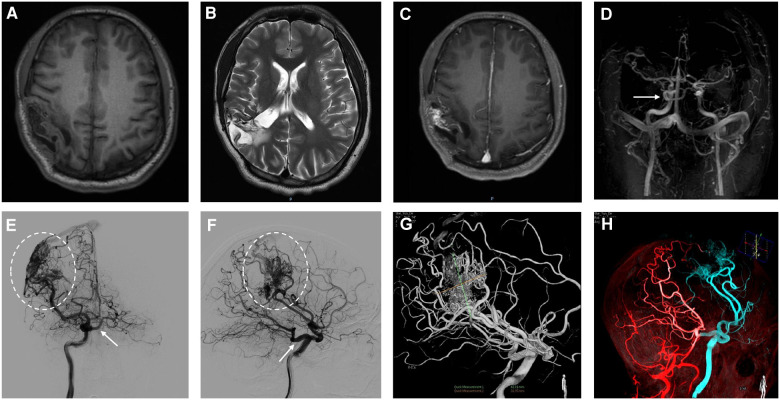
In the right parietotemporal lobe, an irregularly circular space-occupying lesion was found with slightly long T1 shadows (A). T2-weighted image shows a parietotemporal lesion with mixed signal characteristics (B). Enhanced MRI shows heterogeneous enhancement in the right parietotemporal lobe (C). The primitive trigeminal artery arose from the cavernous sinus segment of the right ICA (D). Initial right internal carotid artery injections, anteroposterior (E) and lateral views (F), show shunted flow to the cavernous sinus (arrow). Anteroposterior and lateral projection angiogram shows a right parietotemporal AVM supplied by the right middle cerebral artery drainage into the superior sagittal sinus (E,F, dashed oval). 3-dimensional (3D) DSA arterial phase indicates in the lateral projection showing AVM lesion with 42.8 mm in size and PTA obtained after right internal carotid artery injection (G). Fused image of 3D DSA demonstrates the flow of the right ICA (blue), VA (red) (H).
